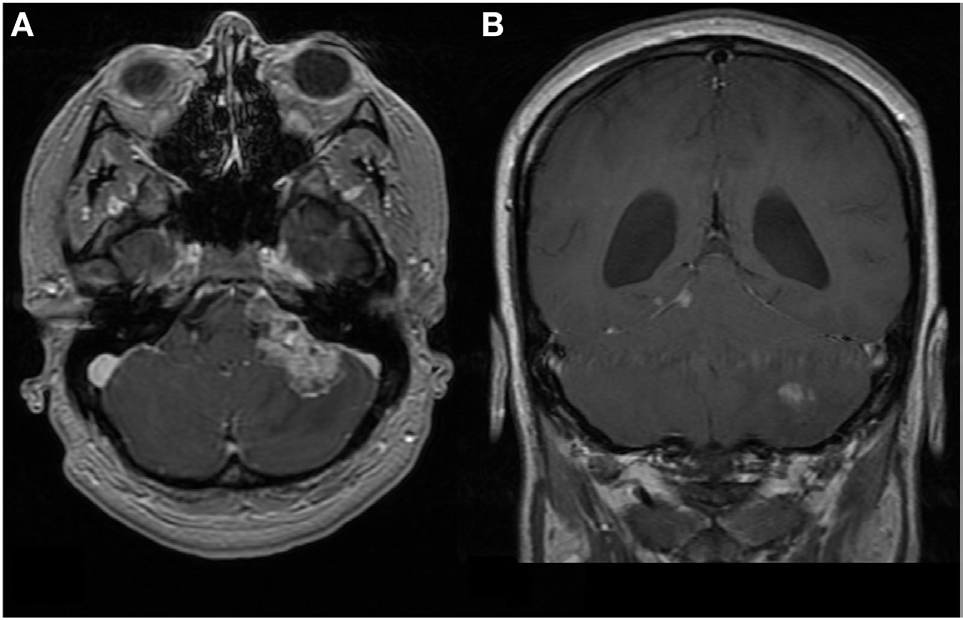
First patient. MRI brain at time of diagnosis, 1.5 T. Images are T1-weighted and Gd-enhanced. (A) Axial: heterogeneous extra-axial enhancement is seen in the left cerebello-pontine angle, with some local compression. (B) Coronal: enhancement is seen along the tentorium cerebelli on the right, which is characteristic of leptomeningeal spread. The left cerebellar lesion appears intraparenchymal.
